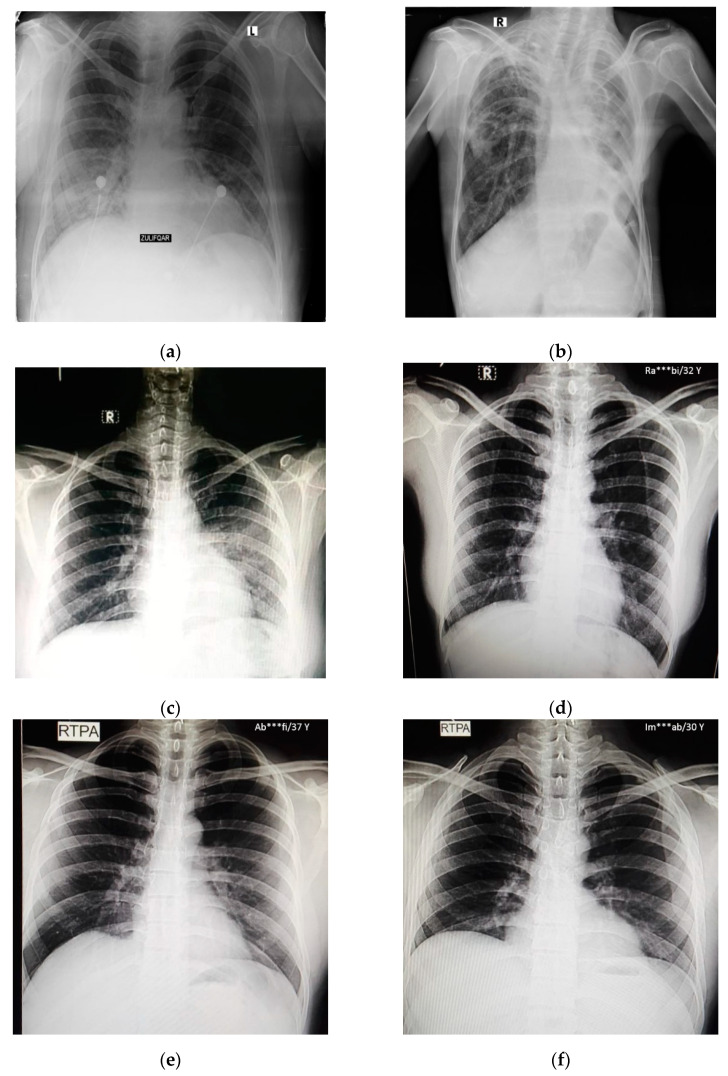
Samples of local Pakistani verified dataset used for the testing of trained models: (a–c) COVID-19 chest X-rays; (d–f) normal chest X-rays.
